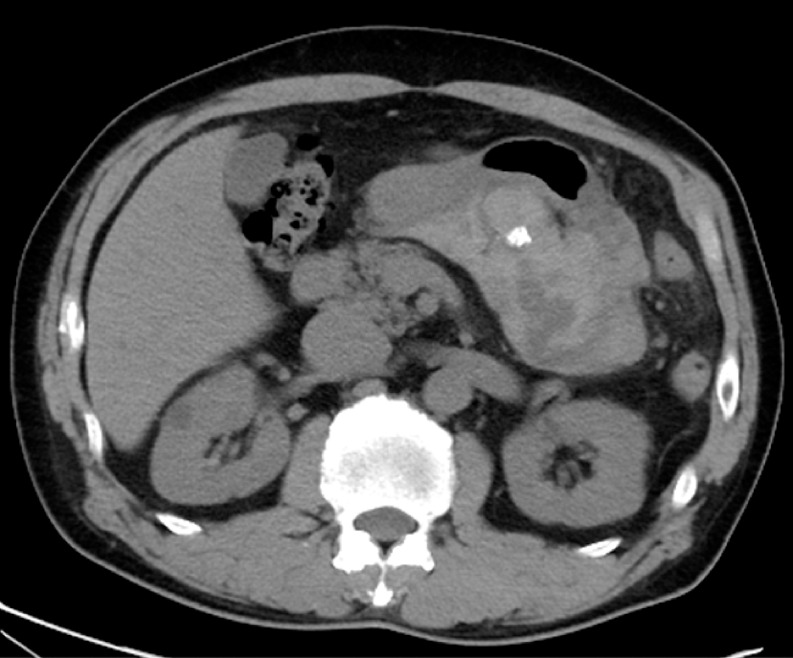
Computed tomography image showing a tumor connected with the gastric wall, with possible perigastric hemorrhage.
